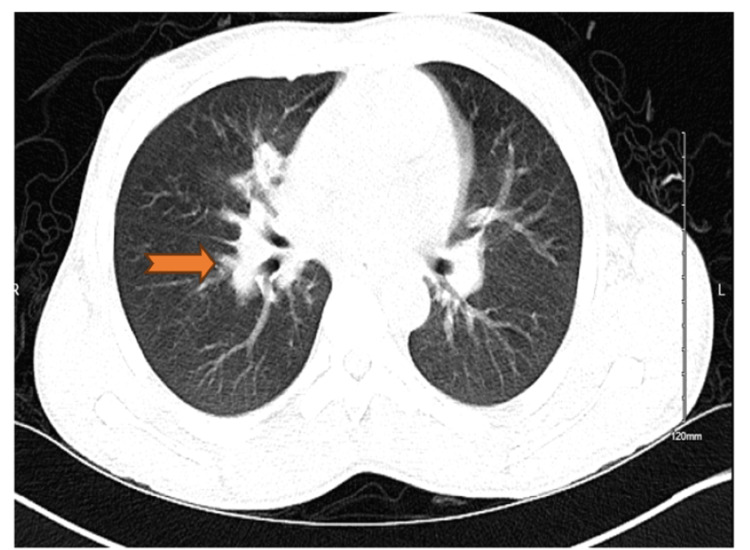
CT scan of the lungs showing right mid-lobe infiltrate
